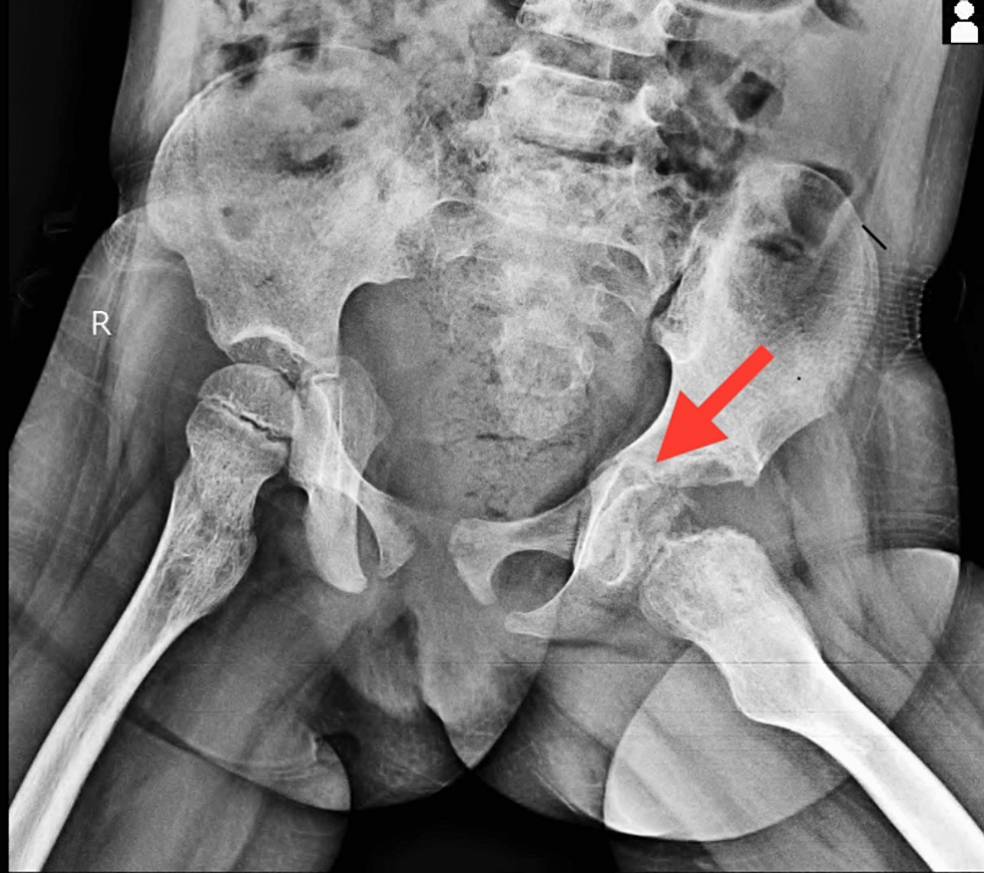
Radiographic imaging of bilateral hip joints (AP view) AP: anteroposterior
